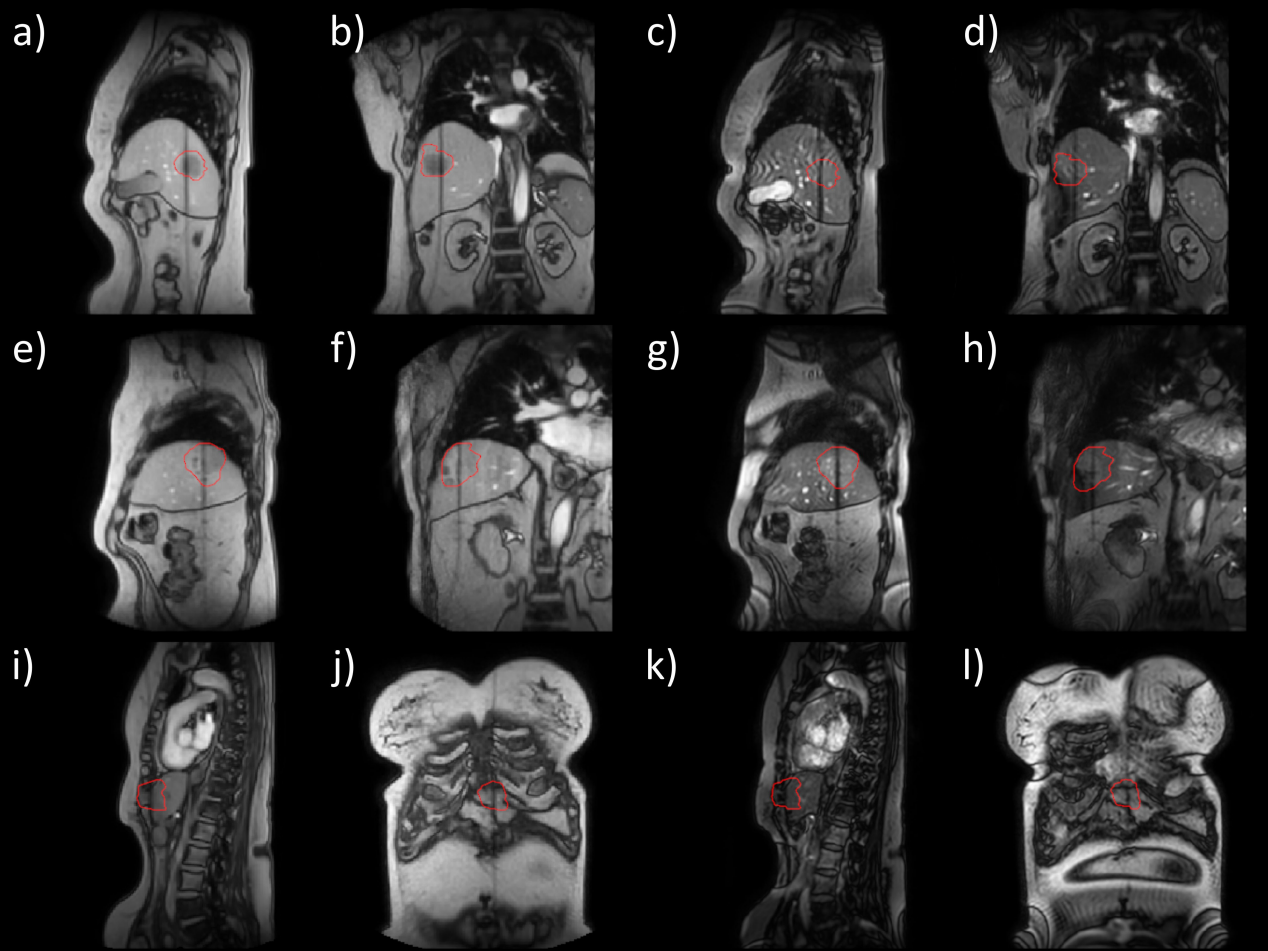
2D cine images captured mid sequence acquisition for liver tumors treated on the Unity for patient 1 (a-d), patient 7 (e-h), and patient 9 (i-l). The shown images are T1-weighted cine MRI sagittal (a, e, i) and coronal (b, f, j), bTFE cine MRI sagittal (c, g, k) and coronal (d, h, l). The target is lightly outlined in red.
